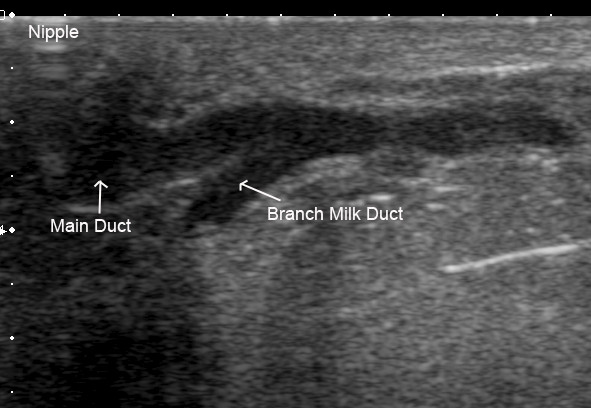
Ultrasound image of a milk duct in a lactating breast. The main duct is 8 mm long and 2.4 mm in diameter. The branch marked is 1.7 mm in diameter. This branch is draining glandular tissue directly under the nipple.
